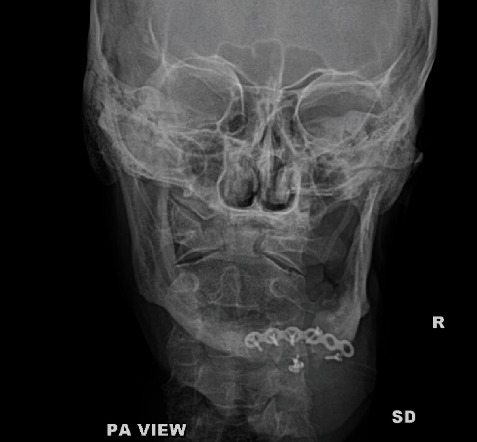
CT scan after hardware failure.
